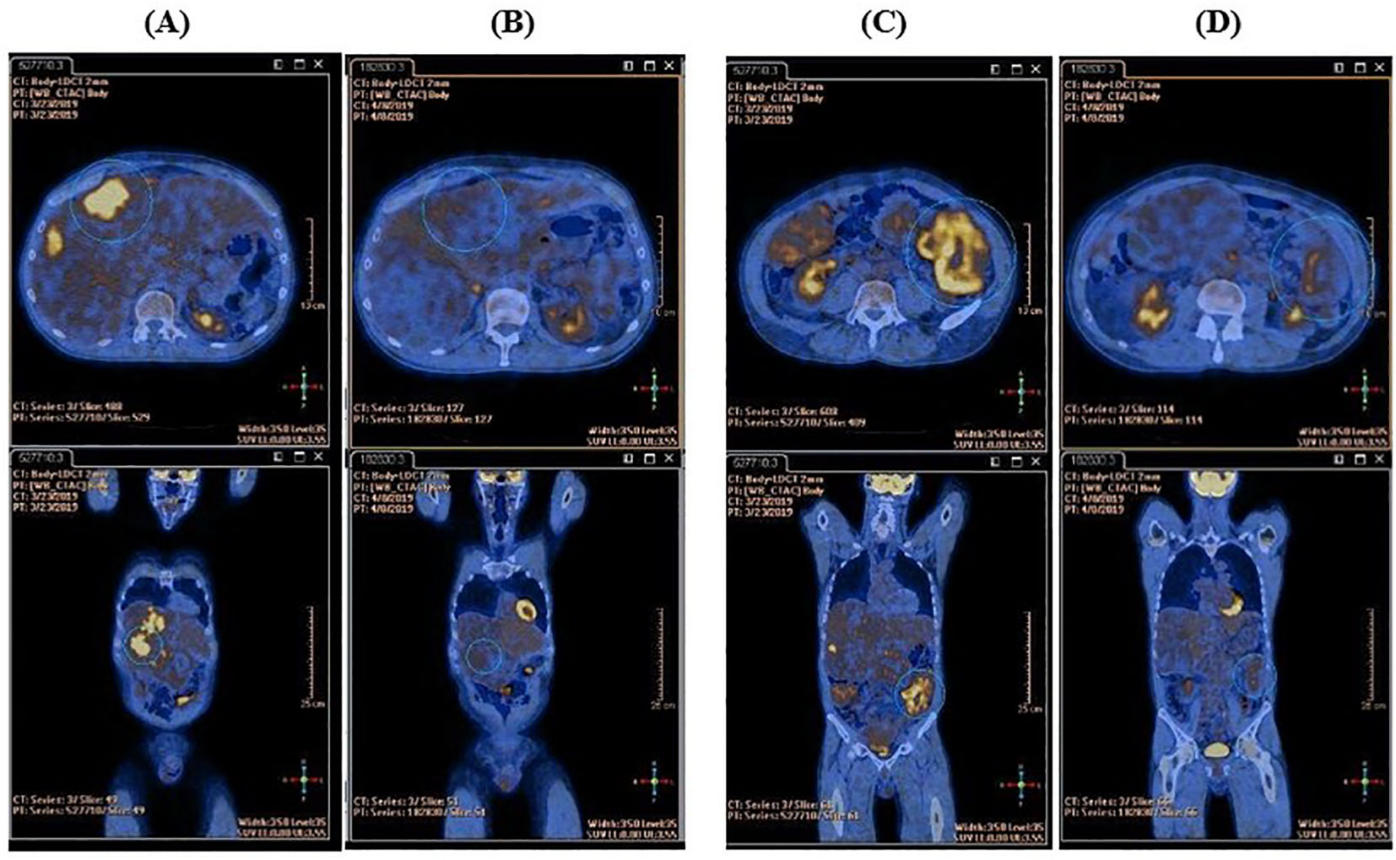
Comparison between the basal FDG-PET and the FDG-PET performed after two weeks of imatinib therapy. (A) High tracker uptake in liver metastasis at baseline. (B) Complete metabolic response in liver metastasis after two weeks of imatinib therapy. (C) High tracker uptake in peritoneal metastasis at baseline. (D) Near-complete metabolic response in peritoneal metastasis after two weeks of imatinib therapy.
